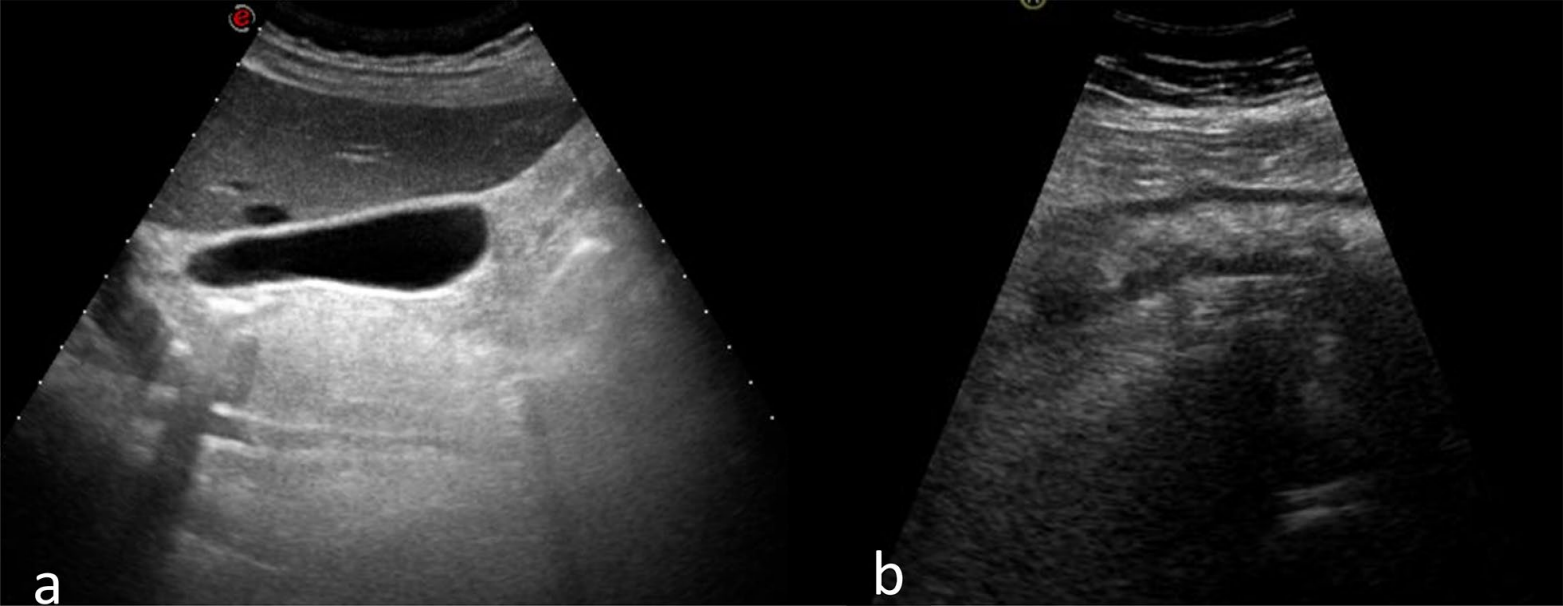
The ultrasound demonstrated normal liver, spleen, kidneys, no free fluid and normal gall bladder (a). A diffuse wall thickening of the bowel in the right upper quadrant (b). The impression was of a right-sided inflammatory bowel disease or colon diverticulitis
